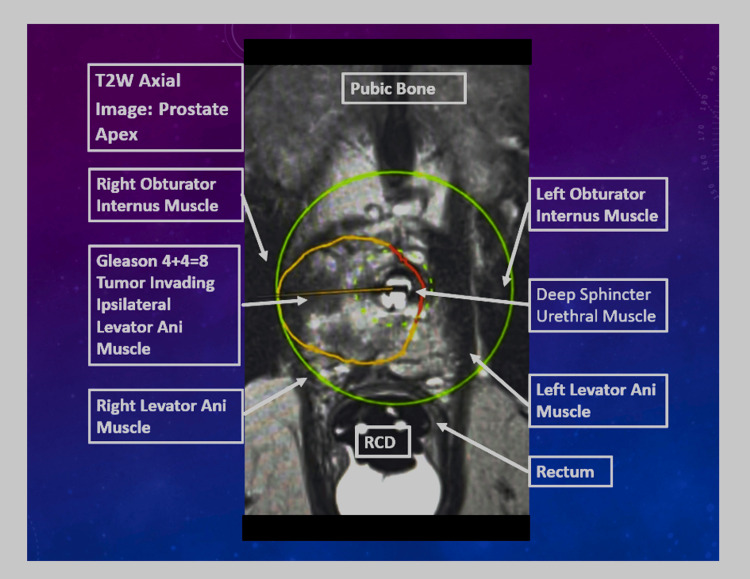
Right-sided Gleason 4 + 4 = 8 apical tumor involving the ipsilateral levator ani muscle Representative axial T2-weighted planning image demonstrating a right apical prostate tumor with extension into the ipsilateral levator ani muscle. The green circle represents the available treatment field. The orange contour indicates the planned treatment zone. The red contour denotes a no-treatment zone designed to preserve the striated urethral sphincter, contralateral levator ani, and obturator internus musculature. RCD: Rectal Cooling Device
